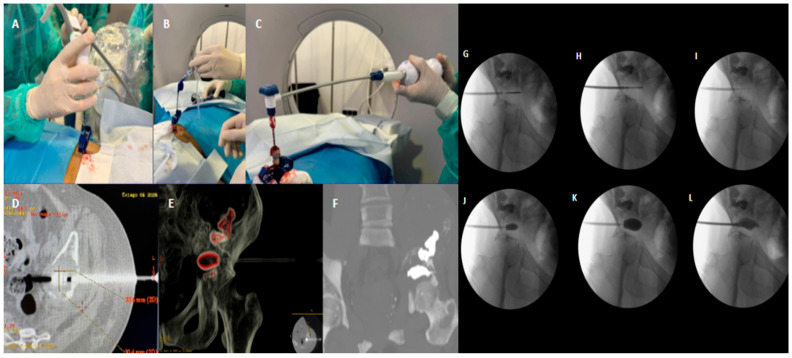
CT/fluoroscopic-guided thermal ablation followed by Balloon-Assisted Acetabuloplasty in a 47-year-old female patient with lung carcinoma and a painful metastatic lesion of the left acetabulum. (A) Sterile preparation of the operative field and initial percutaneous setup at the planned entry site. (B) Fluoroscopic-guided advancement of the coaxial bone access system toward the osteolytic lesion within the acetabular roof, along a safe trajectory to the weight-bearing dome. (C) Insertion of the straight microwave ablation antenna through the coaxial access system into the target lesion. (D) Axial CT image confirming accurate intralesional positioning of the microwave ablation antenna within the osteolytic metastatic focus of the acetabulum. (E) Three-dimensional CT reconstruction depicting lesion morphology and its spatial relationship with the acetabular roof, useful for procedural planning and confirmation of the treatment corridor. (F) Post-procedural CT image demonstrating satisfactory intralesional distribution of polymethylmethacrylate (PMMA) cement within the treated acetabular cavity, with effective structural reinforcement of the load-bearing region. (G) Fluoroscopic image confirming correct positioning of the microwave ablation antenna within the lesion before completion of the ablation phase. (H) Fluoroscopic image showing creation of the intralesional cavity using a drill after thermal ablation, in order to prepare an adequate space for subsequent balloon insertion and controlled expansion. (I) Introduction of the balloon catheter in its deflated state into the previously prepared cavity. (J) Initial inflation of the balloon with contrast medium under continuous fluoroscopic monitoring, allowing progressive cavity expansion and compaction of the devitalized tumor tissue. (K) Full balloon expansion with contrast, obtaining a well-defined cavity within the acetabular dome suitable for controlled cement filling. (L) Final cementation phase under real-time fluoroscopic guidance, showing PMMA injection into the created cavity with satisfactory filling of the lesion and final mechanical stabilization.
